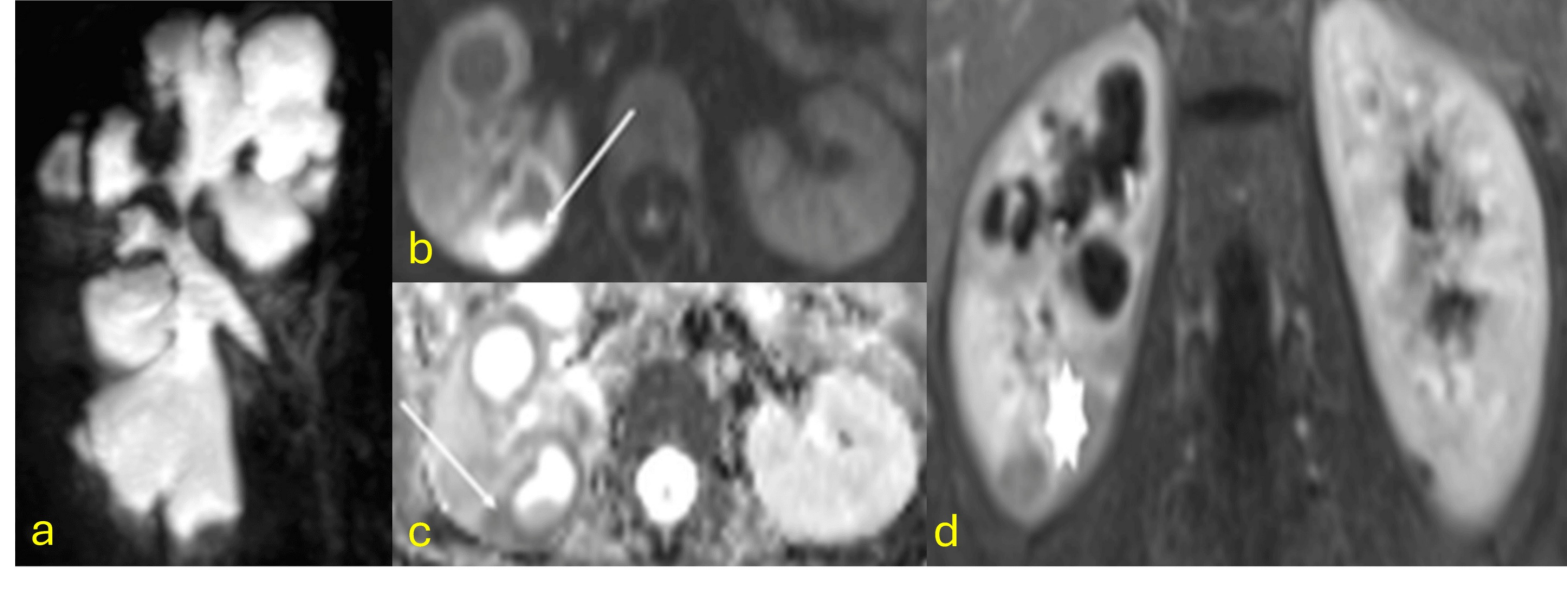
Multifocal infundibular stenosis (a) A patient under evaluation for right hydronephrosis. T2-weighted imaging shows multiple asymmetric infundibular strictures with caliectasis. The ureter is not involved. (b and c) Diffusion restriction is observed in the calyceal walls and dependent contents (arrows). (d) Post-contrast coronal image showing areas of focal pyelonephritis (asterisk). Diagnosis confirmed by urine lipoarabinomannan (LAM) antigen test and positive urine culture for *Mycobacterium ​​​​​tuberculosis*
